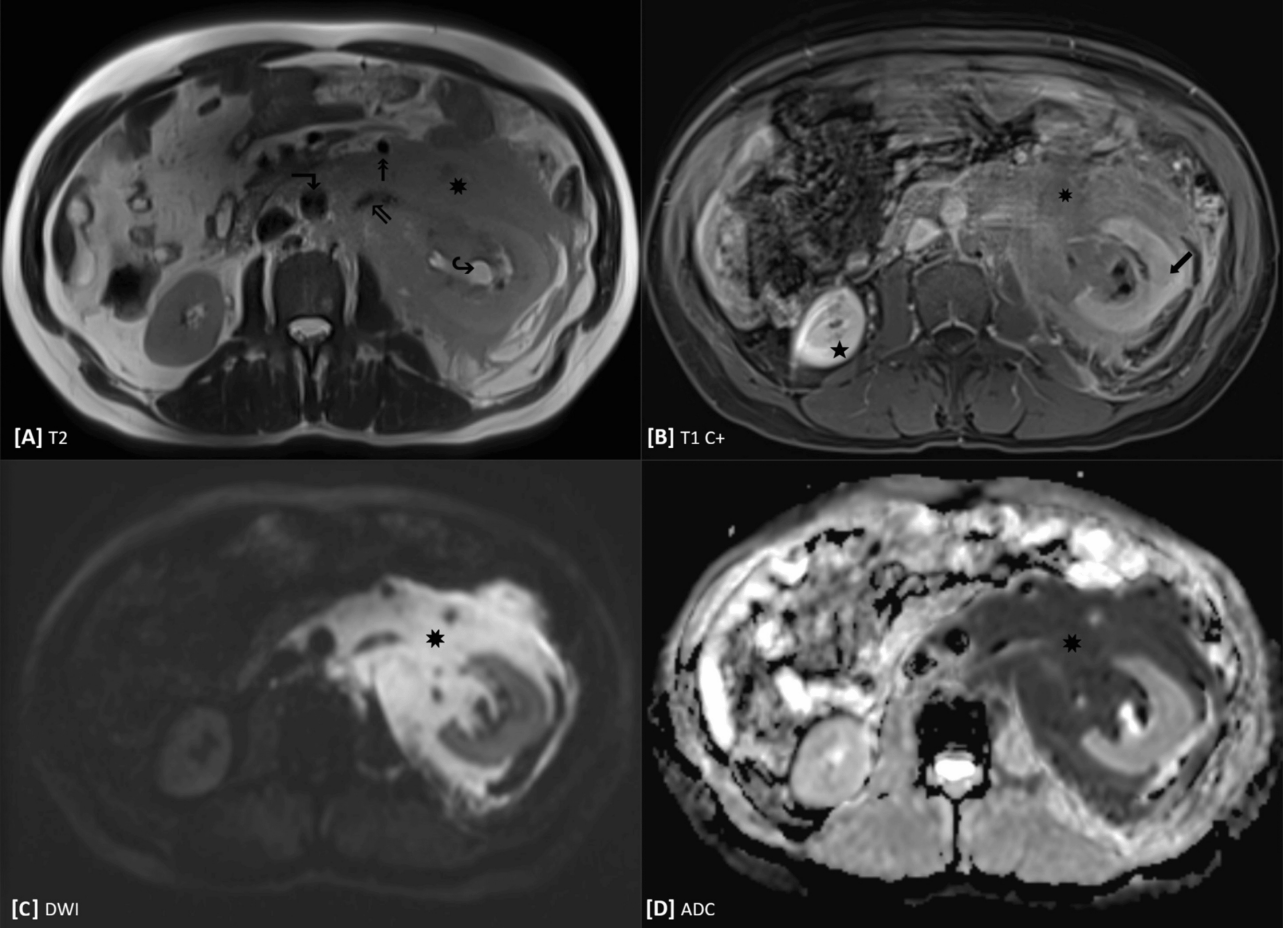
MRI of the abdomen performed with a renal mass protocol. (A) Axial T2-HASTE image redemonstrates a mildly T2-hyperintense left retroperitoneal mass (✸) involving the left perinephric space and extending into the anterior left pararenal space. The mass encases the left renal vein (⇖) with minimal mass effect on the abdominal aorta (↴) and inferior mesenteric vein (↟). Note pelvocaliectasis of the left kidney (↪). (B) Axial post-contrast T1-weighted image demonstrates low-level heterogeneous enhancement of the left retroperitoneal lesion. Again note delayed enhancement of the left renal cortex (⬋) compared with that of the right kidney (★). (C, D) Diffusion-weighted imaging at b = 1000 s/mm² shows high signal intensity within the left perirenal mass, with corresponding low signal on the apparent diffusion coefficient (ADC) map, consistent with marked diffusion restriction and and suggestive of an infiltrative cellular neoplasm.
